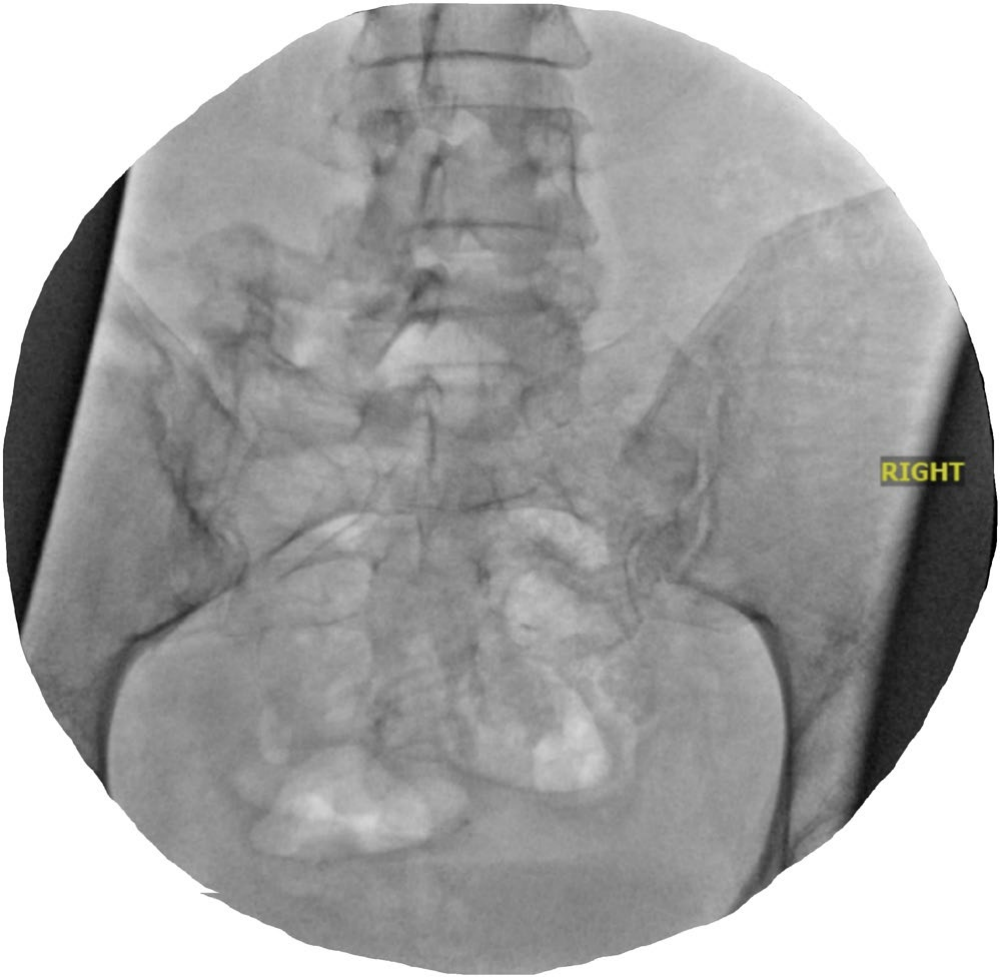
AP radiograph of bilateral sacroiliac joints. Final placement shot of sacroiliac joint stabilizer.
